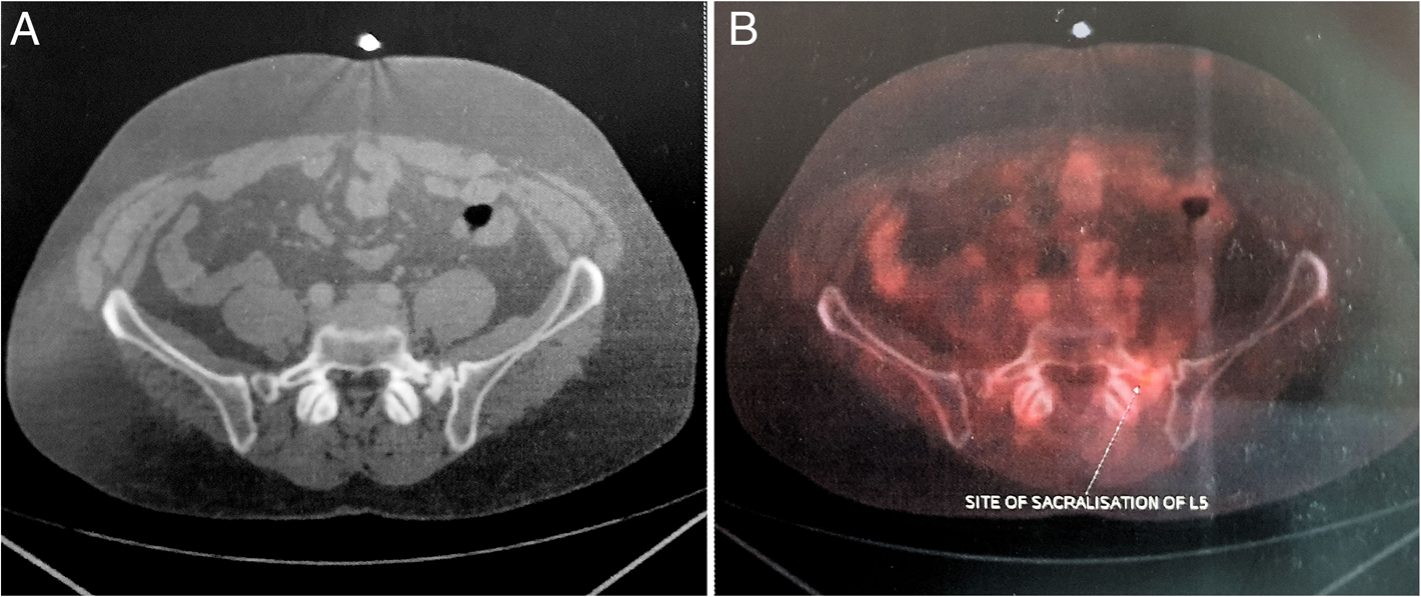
Follow-up FDG-PET in 2019. a Residual sclerosis in the left sacral ala b with the resolution of previous FDG uptake at L5-S1 facet
